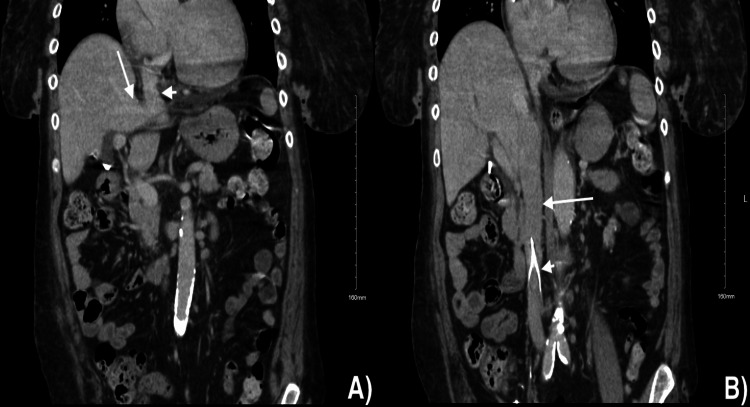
Coronal views of the preprocedural CT of the abdomen. (A) Right hepatic vein (arrow) going into the IVC (arrowhead) at a nearly right angle. (B) IVC (arrow) is patent with the IVC filter (arrowhead) in place IVC: inferior vena cava
